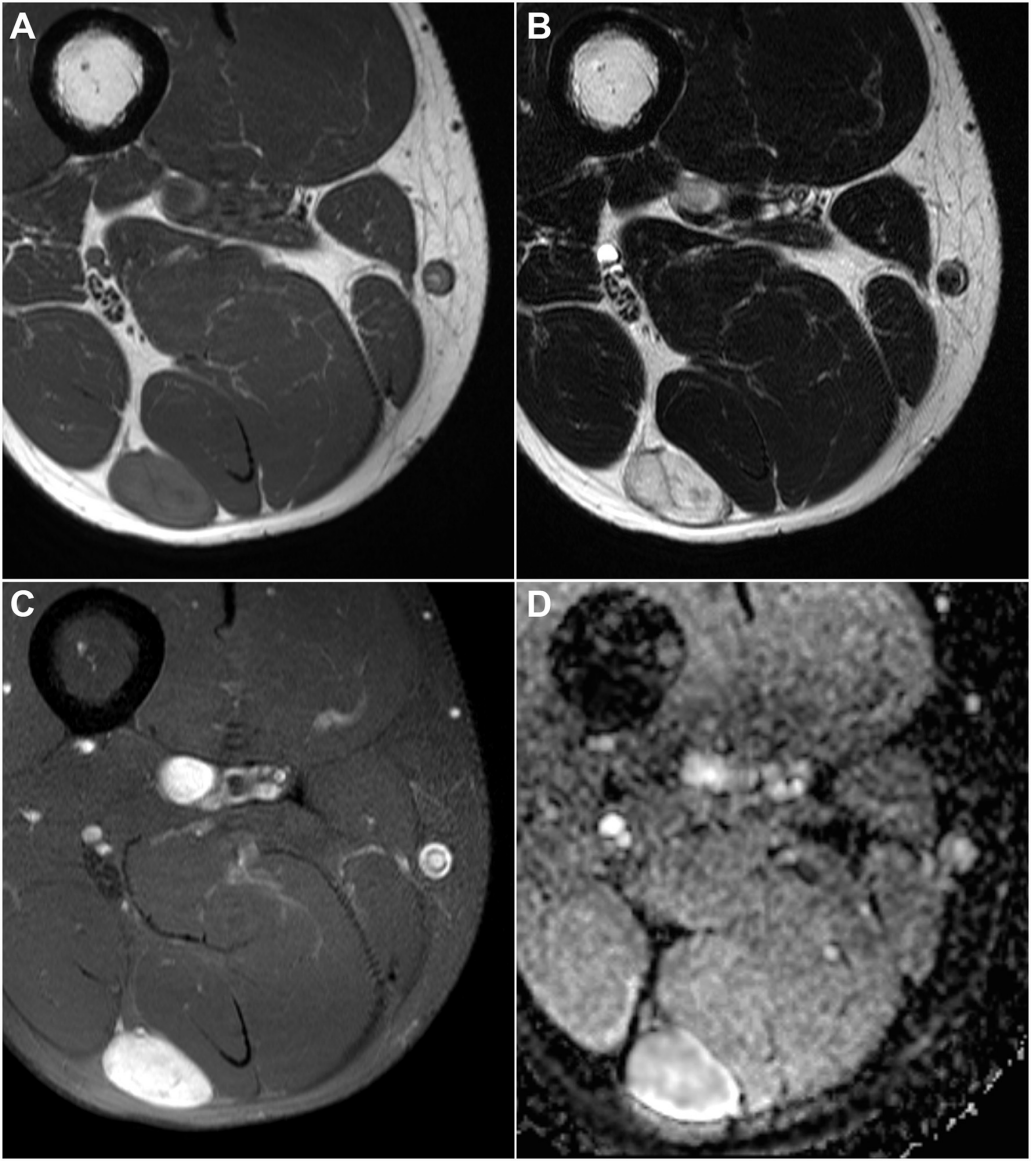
A 44-year-old man with vascular leiomyoma. (A) Axial T1- and (B) T2-weighted images of the right thigh showing a 2.8-cm deeply located oval mass at the intermuscular space of the posterior compartment. (C) Axial fat-suppressed contrast-enhanced T1-weighted image revealed homogeneous strong enhancement. (D) ADC value of the lesion was measured as 1.68 x 10–3 mm2/s. ADC, apparent diffusion coefficient.
